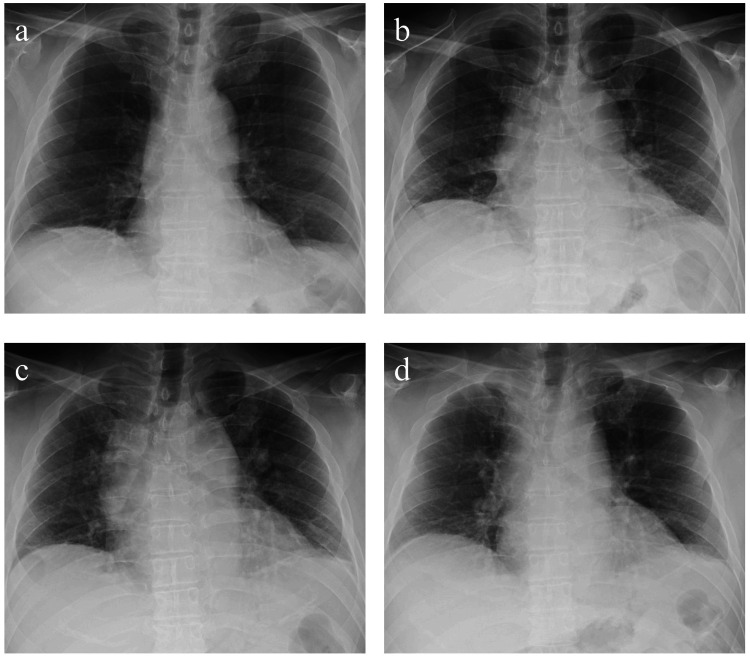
Chest X-ray of the patient on inspiration and expiration. In the upright position, diaphragmatic movements were seen on inspiration (a) and expiration (b), but in the supine position, these movements were absent (c: inspiration; d: expiration).
